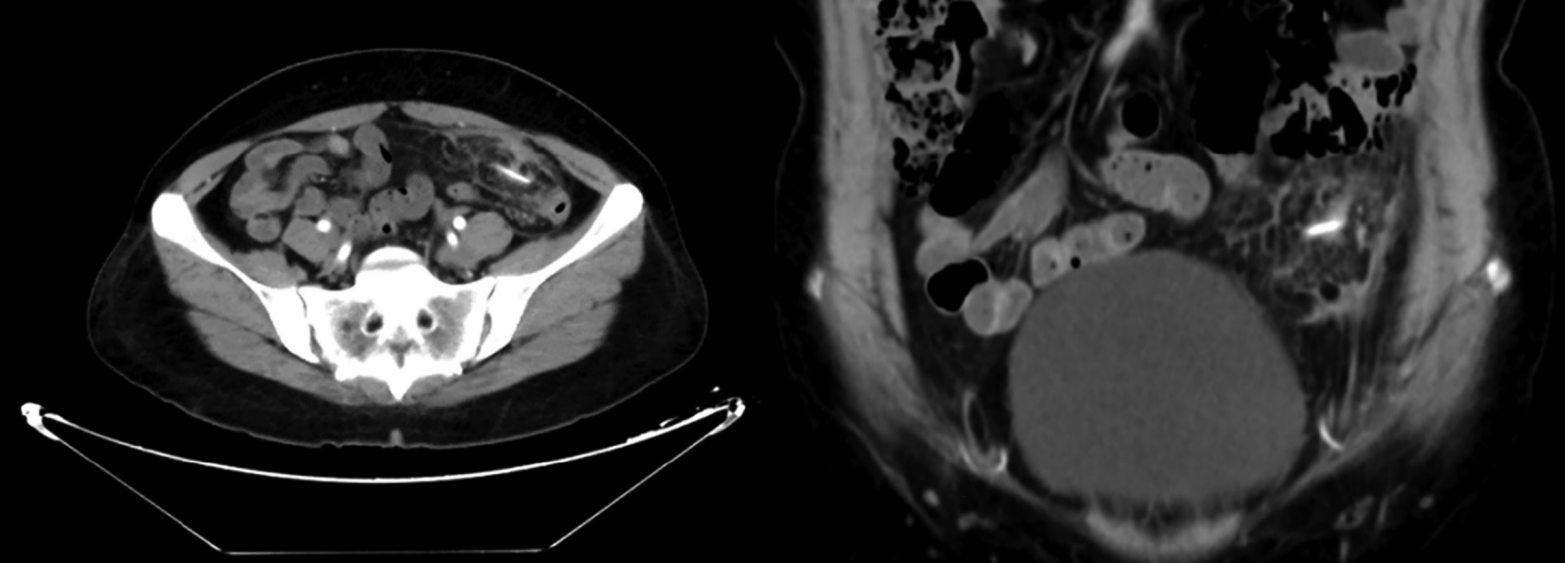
CT scan of the abdominal mass and high‐density lesion.
